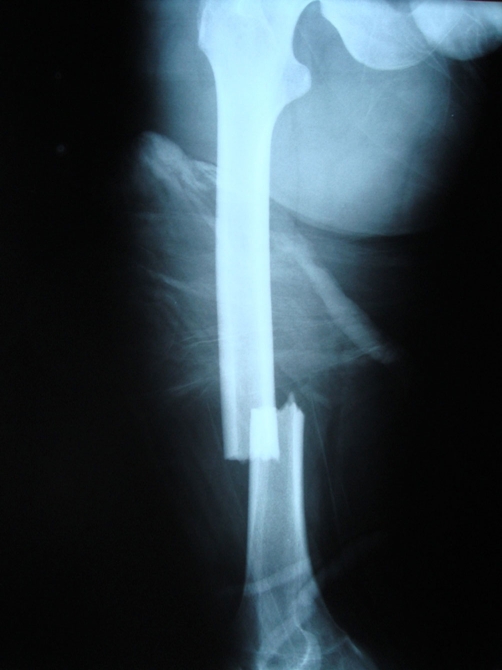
Preoperative AP roentgenography of femur shaft fracture.
